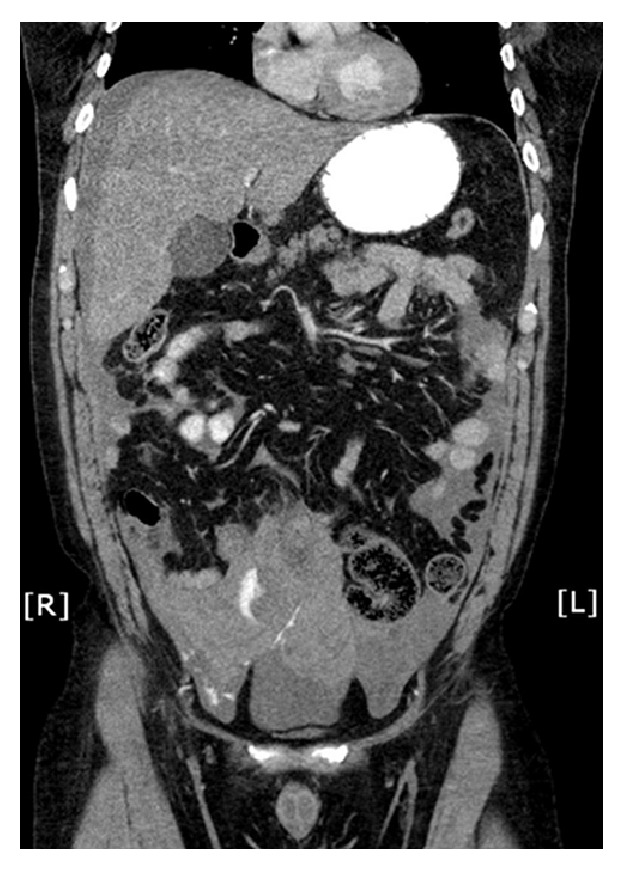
Coronal view of contrasted CT demonstrating the hemoperitoneum with contrast blush.
